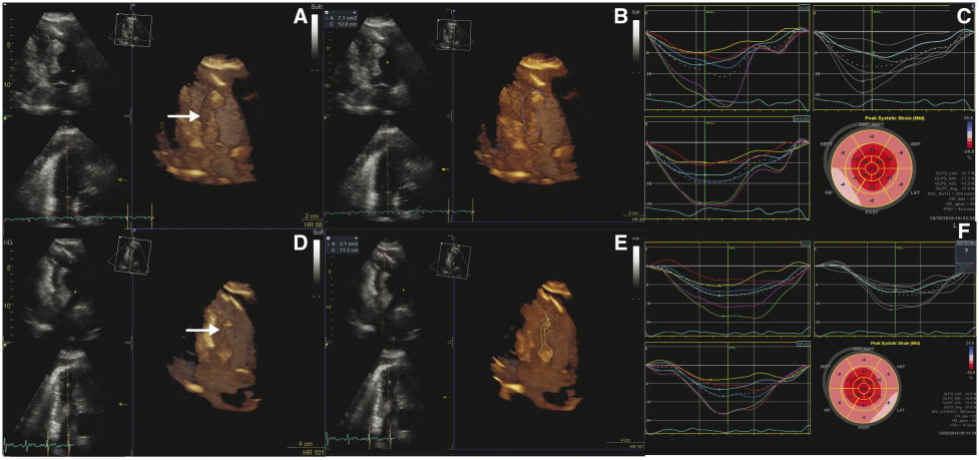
(*A*) Echocardiography before treatment with ipilimumab and nivolumab shows tumour in the right ventricle (arrow) with traced area of 7.1 cm^2^ (*B*). (*C*) Global longitudinal strain was reduced with apical sparing. (*D*) Echocardiography following third treatment with ipilimumab and nivolumab (6 weeks after treatment initiation) shows substantial regression in tumour size (arrow) with traced area reduced to 3.1 cm^2^ (*E*). No improvement in global longitudinal strain was noted at this time (*F*).
